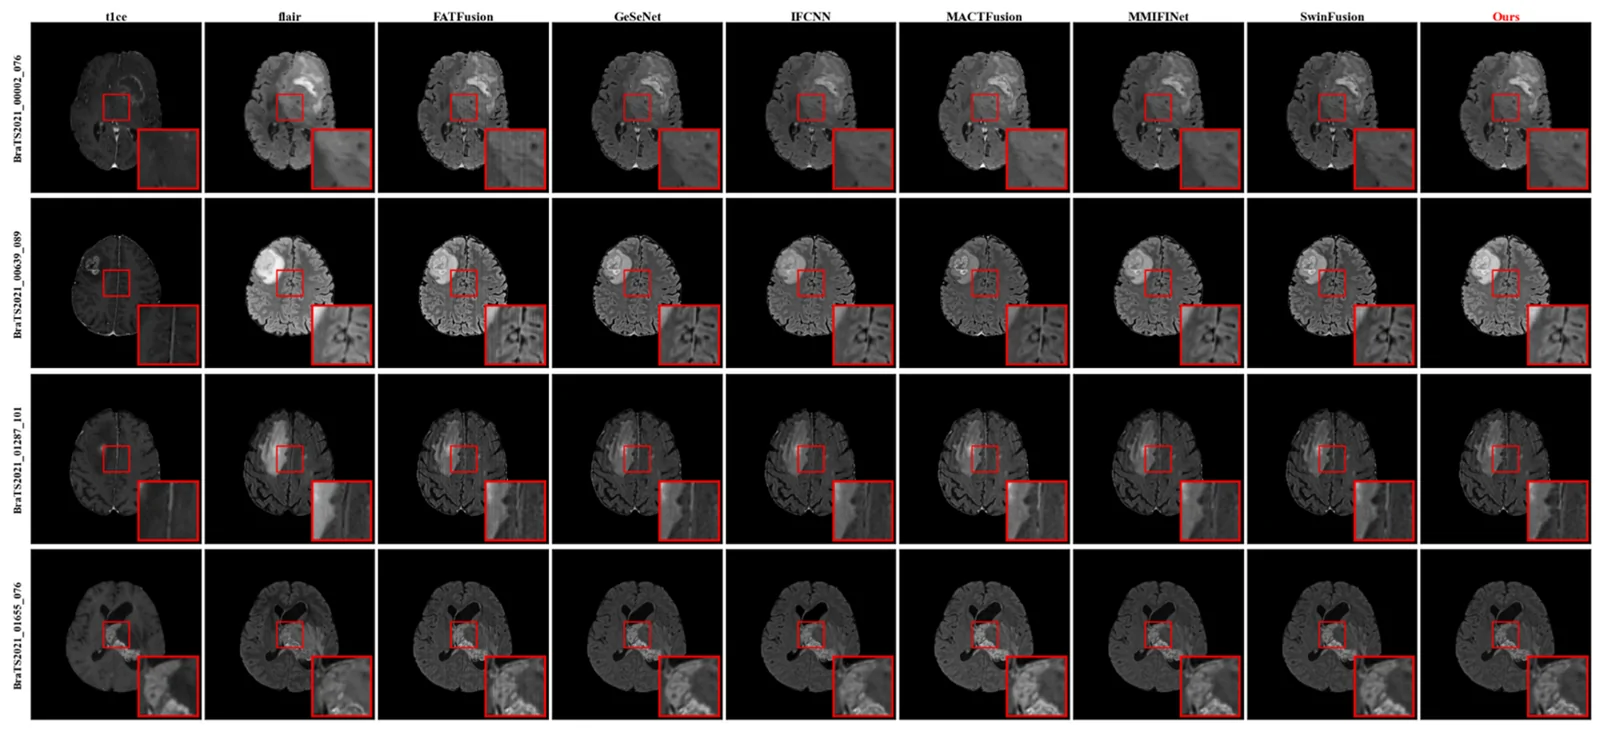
Qualitative comparison of fusion results on the Flair-T1ce dataset. The first two columns display the source T1ce and FLAIR images, respectively. The subsequent columns show the fused results from six state-of-the-art methods (FATFusion, GeSeNet, ECNN, MACTFusion, MMIFINet, and SwinFusion) alongside our proposed MSFusion (ours). The red box indicates local zoomed-in details.
